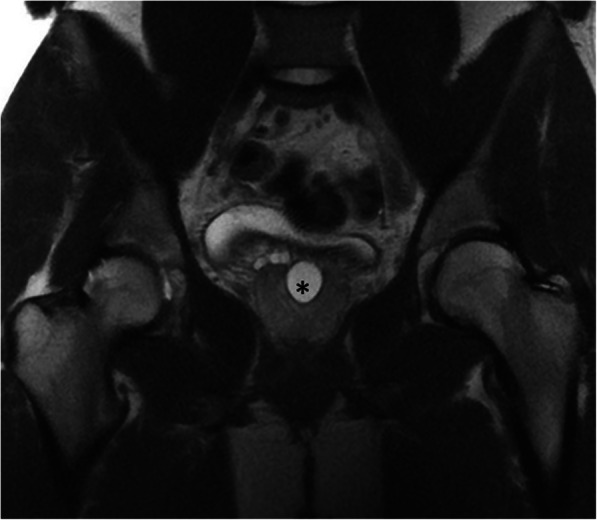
A 17-year-old male patient with urinary incontinence. Coronal plane T2W MR image shows a midline prostatic cyst (asterisk) that does not extend above the prostate gland
